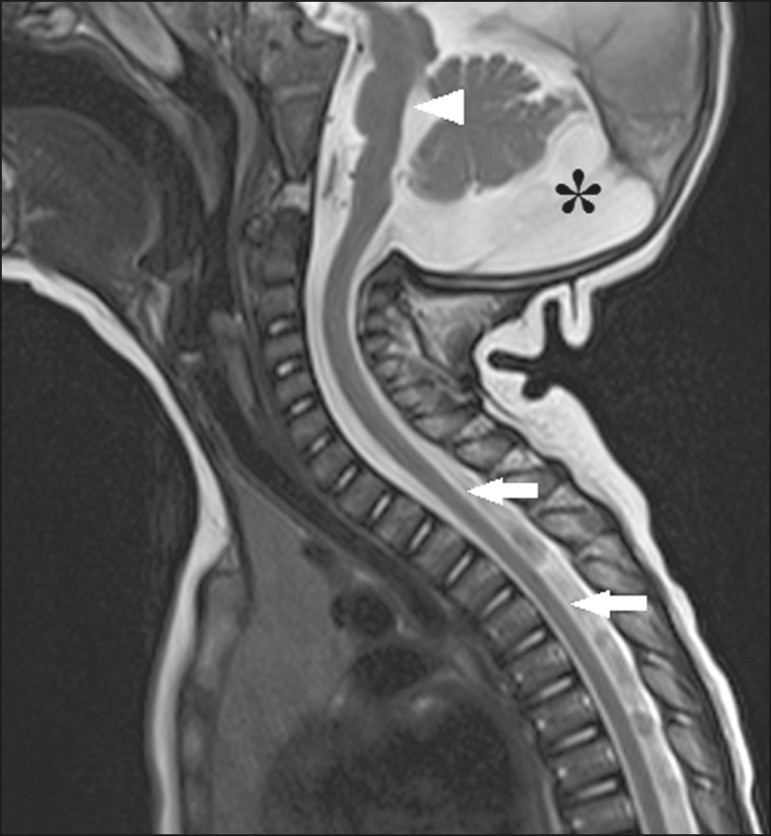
A 14-month-old patient. Sagittal T1-weighted MRI slice showing tapering
